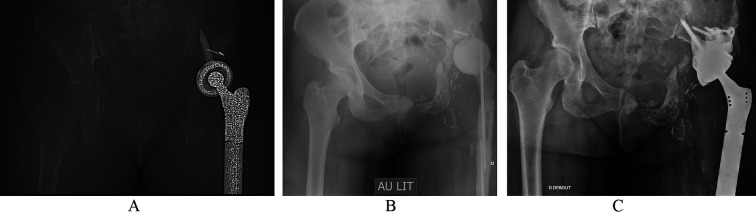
Second case of Hip chronic PJI. Female, history of osteosarcoma of the acetabulum 11 years ago. Pathogen: *Streptococcus agalactiae*. (A) Pre-operative AP X-ray. (B) Preformed articulated spacer. (C) 2-year follow-up X-ray.
